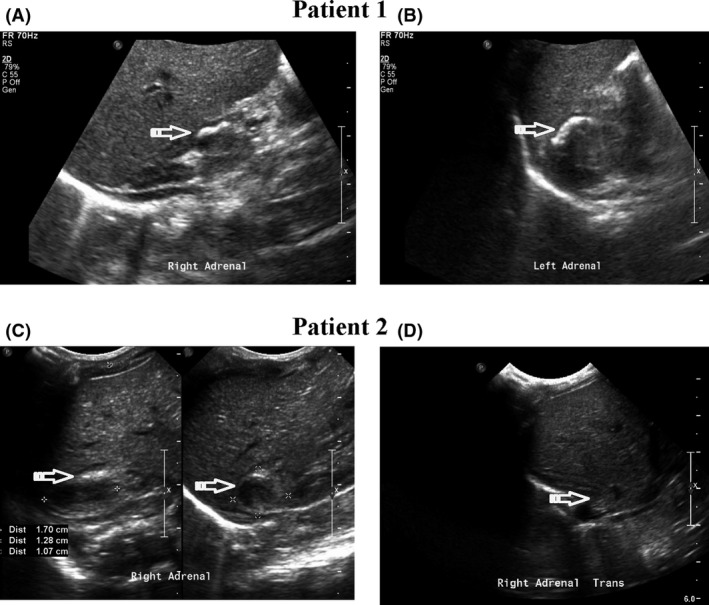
Patient 1: 39‐week GA newborn with bilateral calcifications, low baseline cortisol level, and adequate cortisol response after ACTH stimulation. (A) and (B) are ultrasonographic images of the right and left adrenal showing in part cortical calcifications. Arrows show the calcified areas. Patient 2: 35‐week GA newborn, with right adrenal hemorrhage, abnormal bleeding studies, low baseline cortisol level, and inadequate cortisol response after ACTH stimulation. (C) and (D) are ultrasonographic images of the adrenals of the same patient 7 days apart and show resolution of the hemorrhage. Arrows show the adrenal area of interest.
